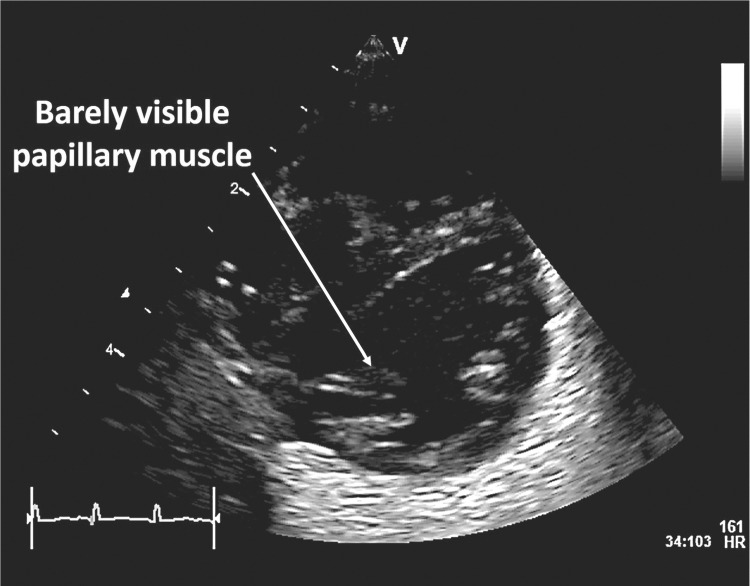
An example of beam width artifact in the parasternal short axis view at the level of the papillary muscle
